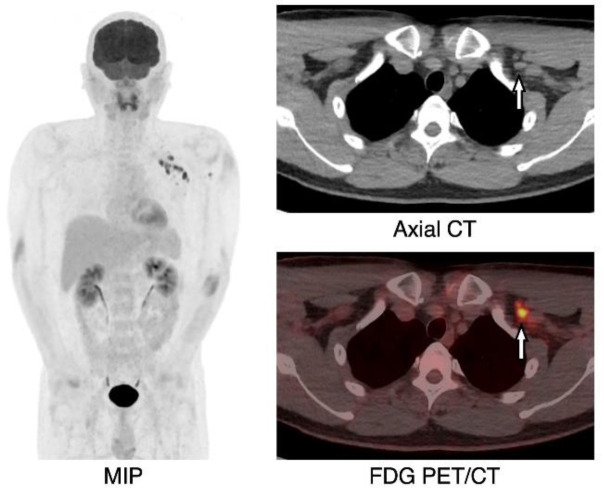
A 49-year-old male, FDG PET/CT (MIP) showed multiple FDG uptakes in 12 left axillary lymph nodes from levels 1 to 3 (SUV_max_=5.9–10.3). Axial CT and FDG PET/CT showed the lymph nodes that were the largest in this case (arrow, short axis: 6 mm, SUV_max_=10.3)
